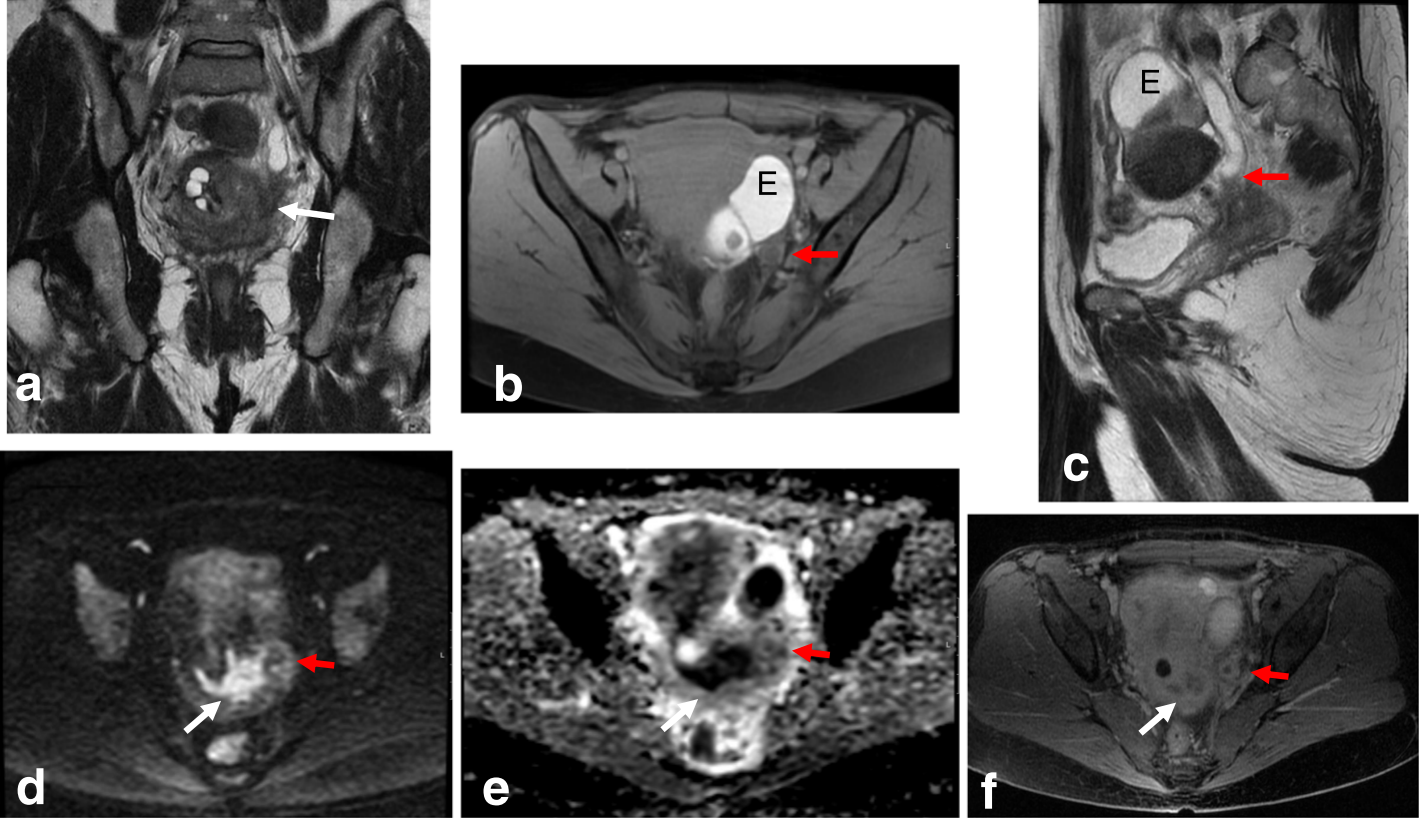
MRI reveals FIGO IIIB cervical cancer in a 38-year-old woman with adenomyosis and endometriosis in the left ovary. Visualization by coronal T2WI (a), axial FS T1WI (b), and sagittal T2WI (c) reveals a mass extending to the left parametrium and involving the left ureter (red arrows) below the endometrioma (E), with a subsequent hydronephrosis. d, e DWI (*b* = 1000/ADC map) reveals diffusion restriction of the cervical mass, with extension to the left ureter. f DCE sequence shows enhancement of the cervical mass and ureter wall
